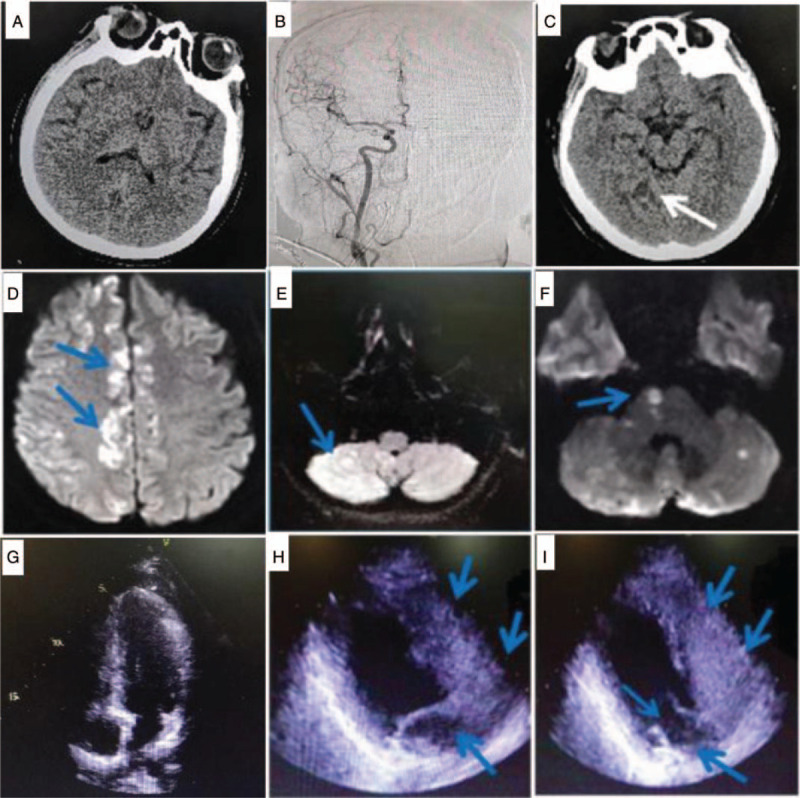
The imaging examination. A, An emergency CT examination was performed. No obvious abnormality was found in the density of brain parenchyma. B, DSA angiography showed no obvious abnormality in the whole cerebral blood vessel network (the image is the angiography image of the right carotid system). C, CT was rechecked 2 days after surgery, and the density of brain parenchyma was found to be uneven. On the right side, new patchy and slightly low-density shadows are seen in the basal ganglia and temporal occipital lobe. On the 5th day postsurgery, MR showed multiple lesions in bilateral brain, cerebellum, and brainstem, more pronounced on the right side; DWI, Diffusion Weighted Imaging images showed multiple spot-like, patch-like, line-like, and nodule-like high signal shadows in the bilateral cerebrum (D), cerebellum (E), and brainstem (F), more pronounced on the right side. G, The echocardiography reexamined after the operation showed that the structure of the heart was basically normal, no signal of left to right shunt was detected in the middle of the atrial septum; no echo interruption was detected, the ventricular septum was continuous and complete, and there was no sign of patent ductus arteriosus. H, Contrast echocardiography of the right heart. 10 days after the operation, normal saline contrast agent (agitated saline mixed with air) was injected by left elbow vein, the images of the right atrium and right ventricle were sequentially completed. And a small amount of contrast signal (blue arrow) appeared in the left atrium and left ventricle after 3 cardiac cycles. I, The above process is repeated twice, with identical results: in the image, the mitral valve has opened, and a small amount of contrast signal has entered the left ventricle. DSA = digital subtraction angiography, DWI = diffusion weighted imaging, MR = magnetic resonance.
